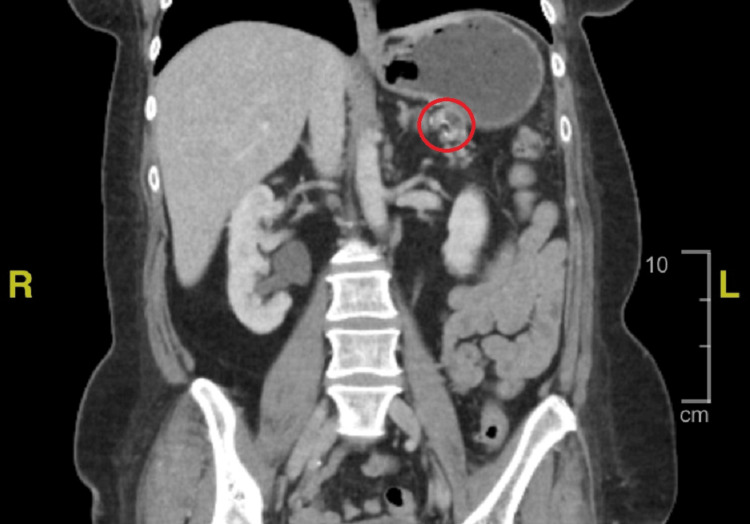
Representative image of a CT scan of the abdomen after splenectomy showing an absent spleen and a filling defect indicating thrombus in the ligated splenic artery. Area within the red circle indicates the location of the thrombus.
